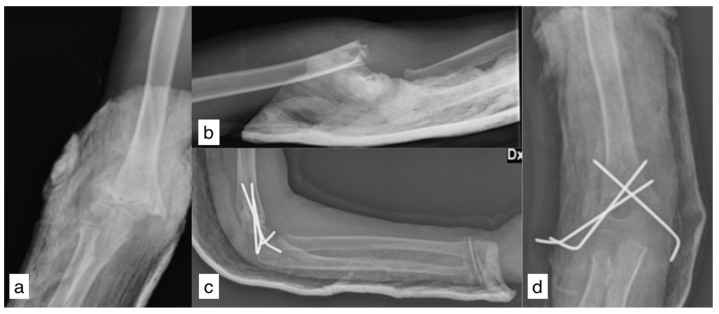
Pre-operative X-rays (a,b); postoperative X-rays (c,d).
